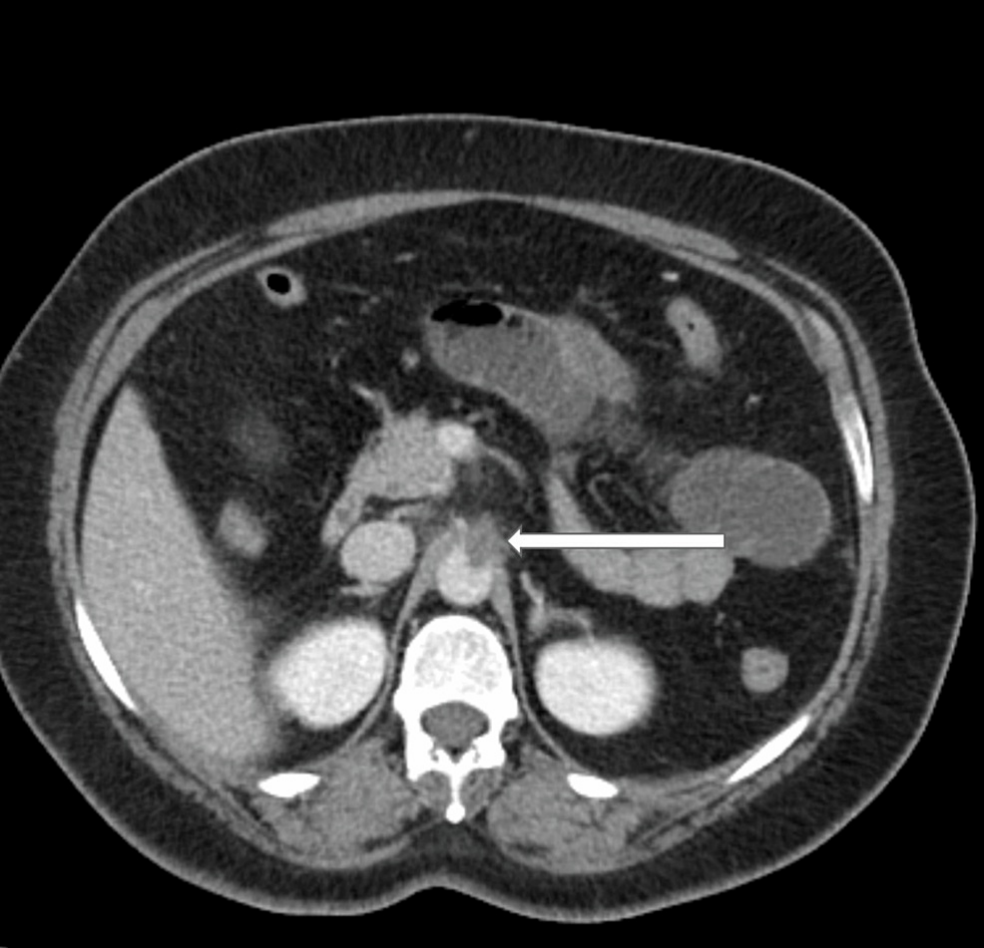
Abdominal CTA image ( axial view) showing abdominal aortic anterior mural thrombus centered on the origin of the celiac trunk artery (white arrow)
